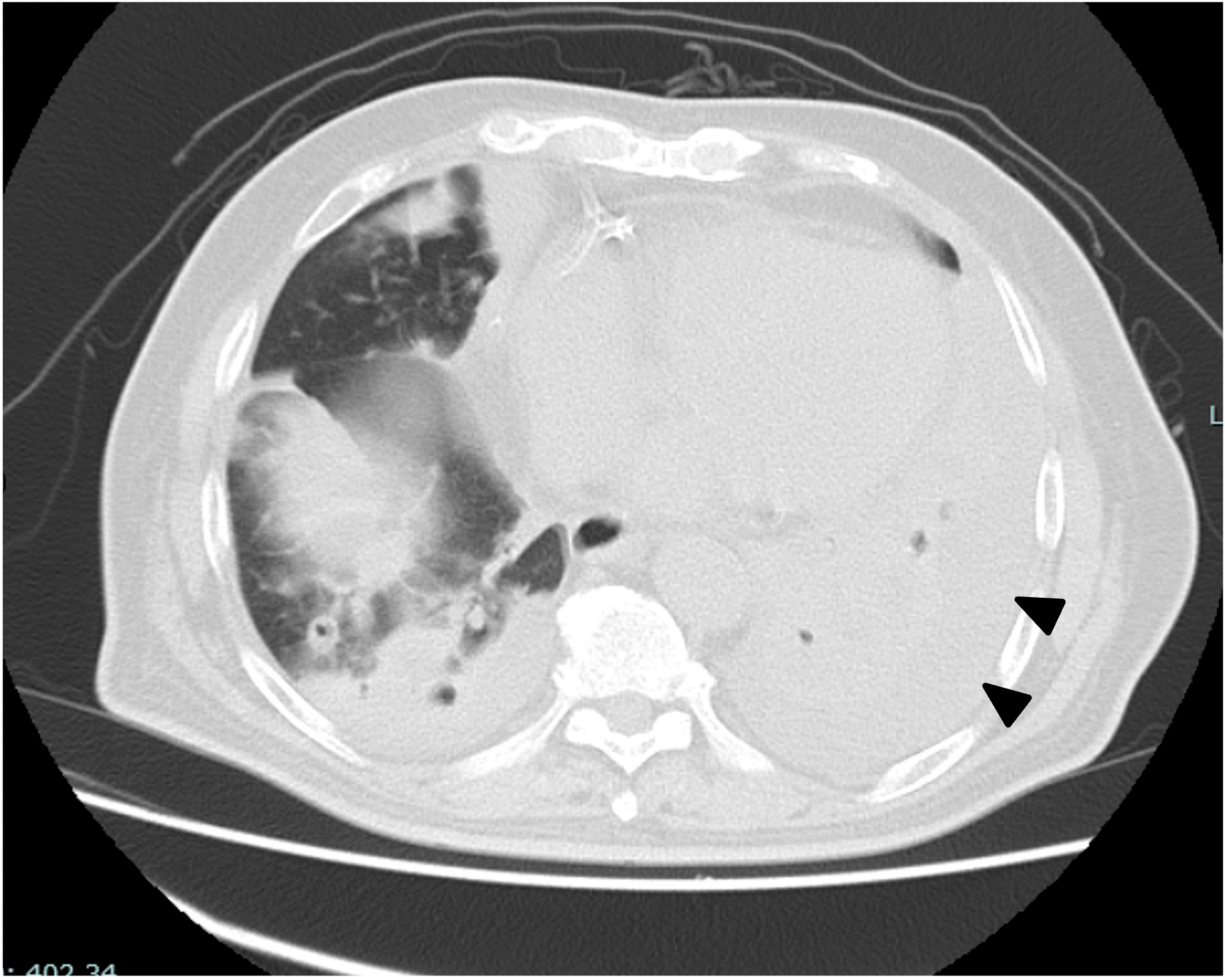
Chest computed tomography scan on Day X+21 demonstrating a reduction in the size of the cavitary lesions and pleural effusion occupying the thoracic cavity. Additional infiltrative opacities are observed, suggesting concomitant pneumonia.
